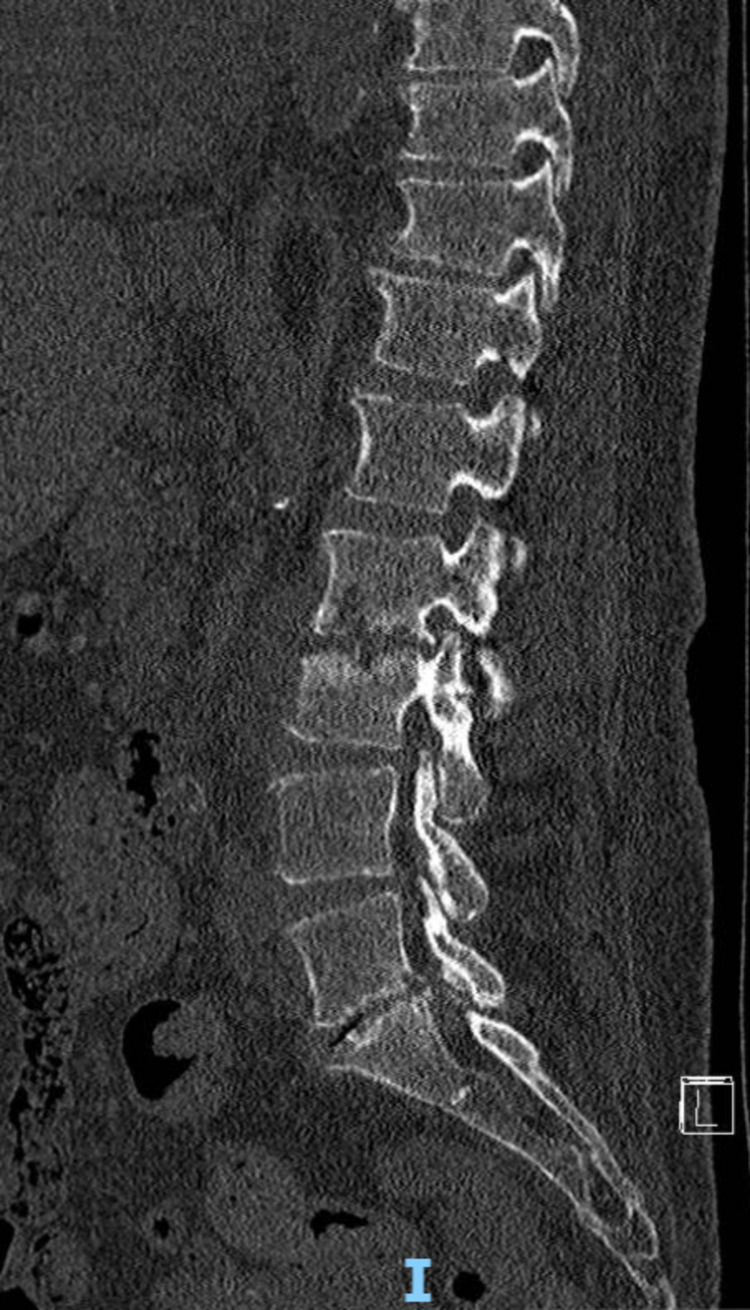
Sagittal CT view of the lumbar spine with changes in L2L3 and L5S1 endplates
